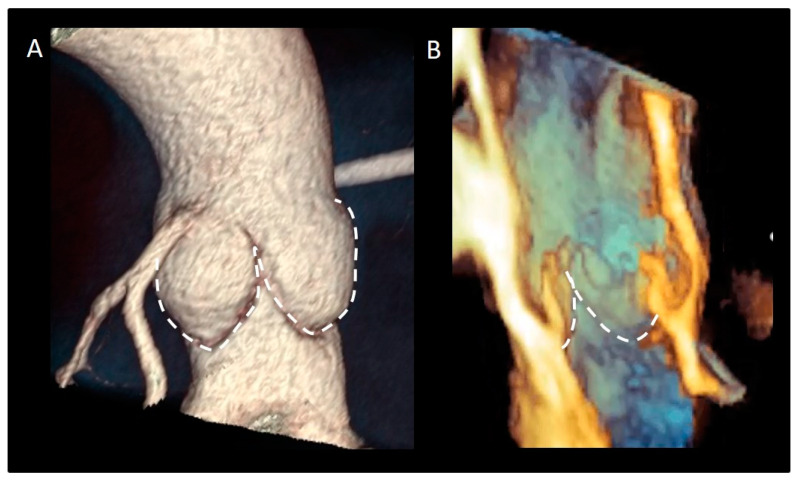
(A) CT in volume rendering format and (B) 3D TEE showing the typical crown-shaped configuration of the anatomical annulus (dotted line).
